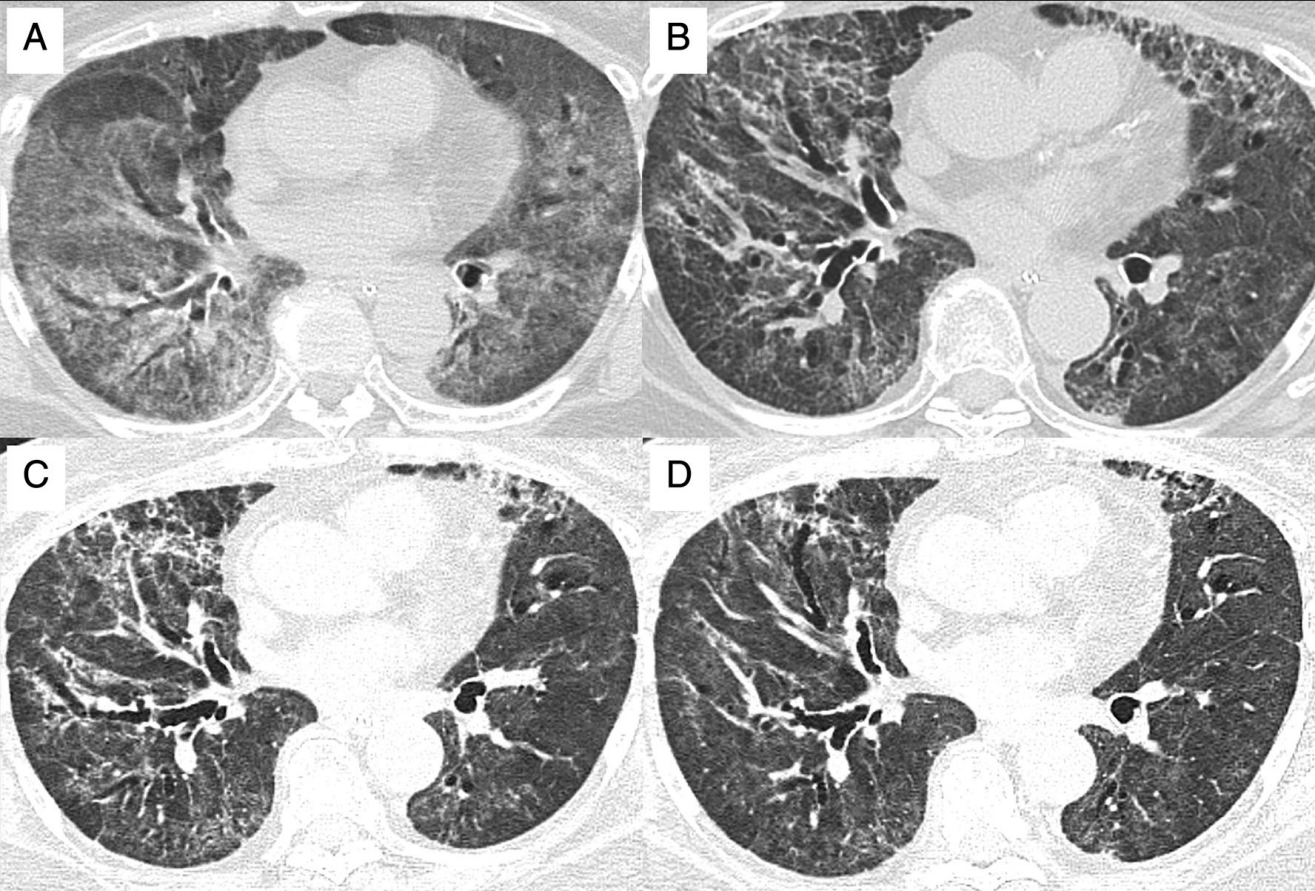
Computed tomography images of the chest (A) when requiring mechanical ventilation; (B) two weeks after extubation, requiring high‐flow nasal cannula oxygen therapy and nasogastric tube feeding; (C) when initiating nintedanib therapy; and (D) two months after the implementation of nintedanib treatment.
